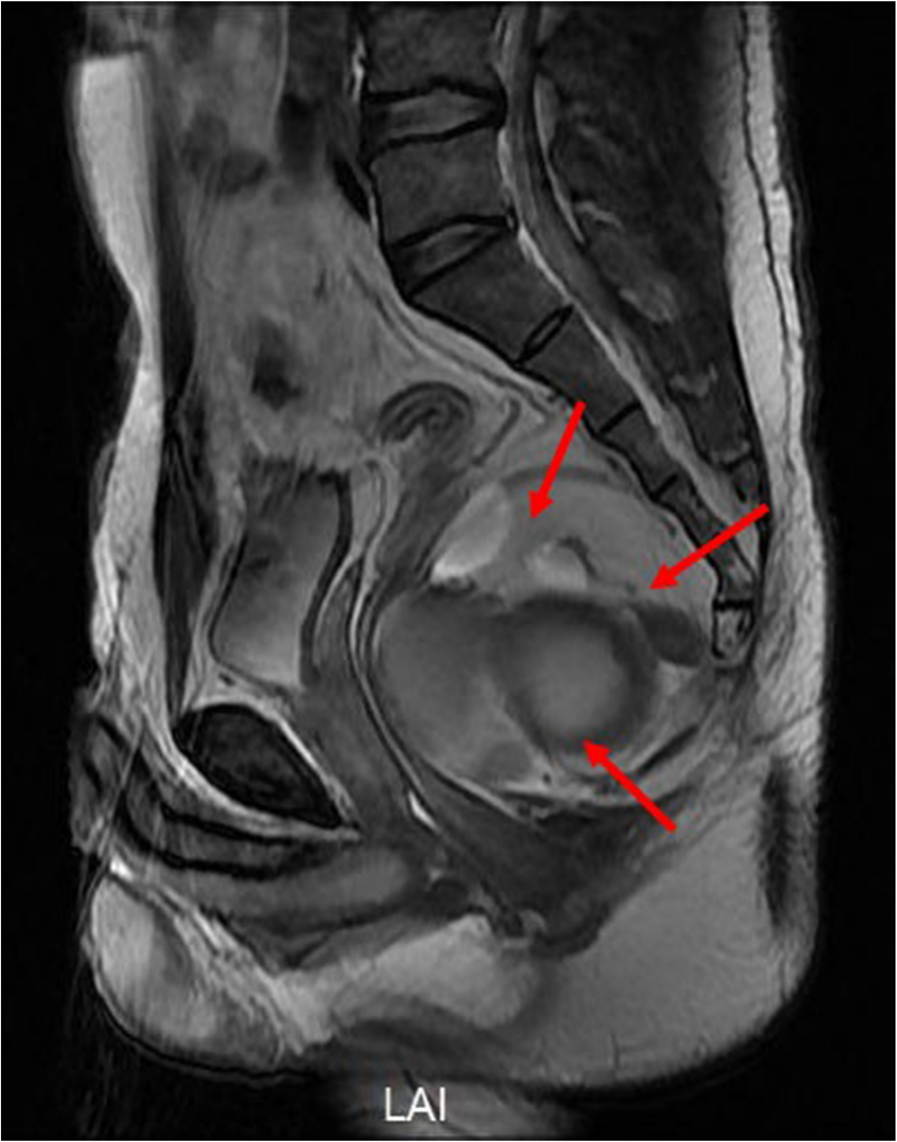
MRI examination of the tailgut cysts. Red arrows indicate the tailgut cysts
